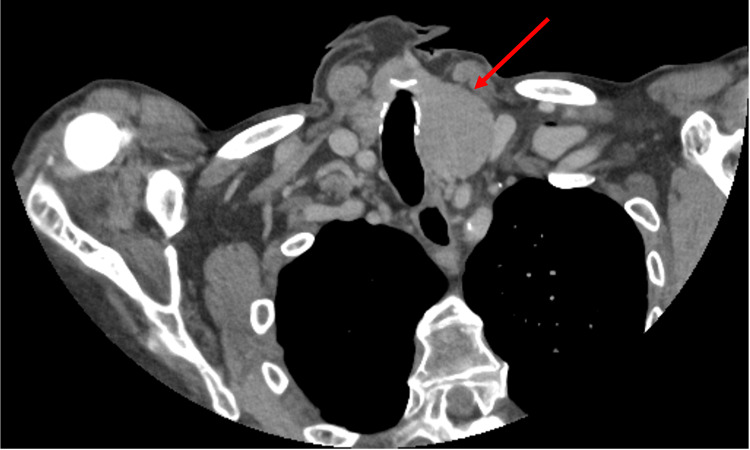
Contrast-enhanced CT scan of the neck performed five months after the diagnosis of GD An enlarged left thyroid lobe with contralateral tracheal deviation is demonstrated (arrow). GD, Graves’ disease
